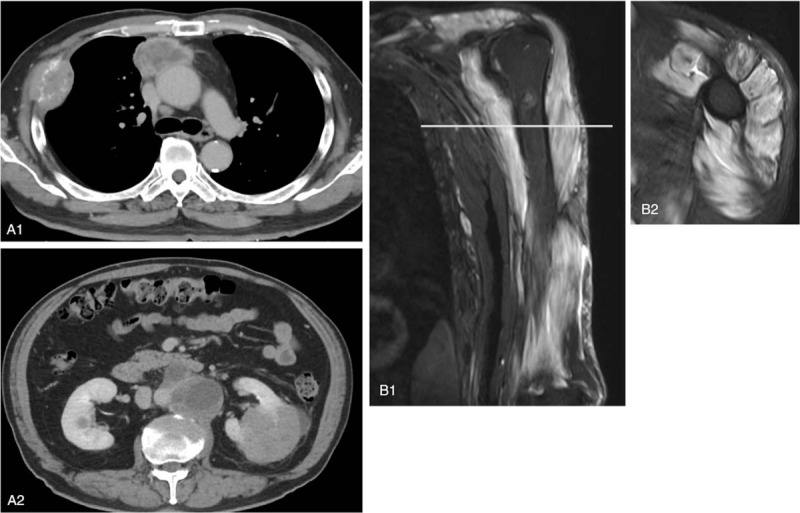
(A) Truncal contrast-enhanced computed tomography at the diagnosis of multiple tumors. (A1) Tumors of mediastinal lymph nodes and the right thoracic wall. (A2) Tumors of the left kidney and para-aortic lymph nodes. (B) Short-tau inversion recovery MRI showing broad hyperintensity in left upper limb muscles. (B1) Coronal section. (B2) Axial section.
